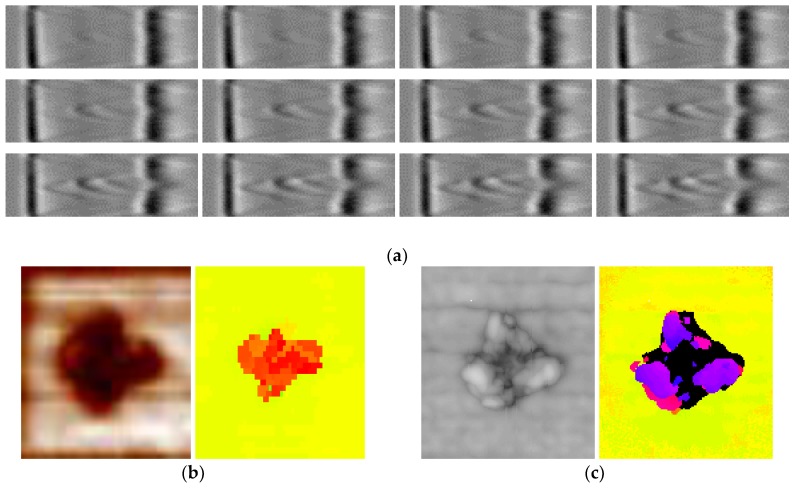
Ultrasonic testing (UT) results: (a) the representative set of UT B-Scans (nos. 54÷65 from 170 in total); amplitude and Time-of-Flight (ToF) C-Scans obtained using (b) phased-array, and (c) pulse-echo methods.
